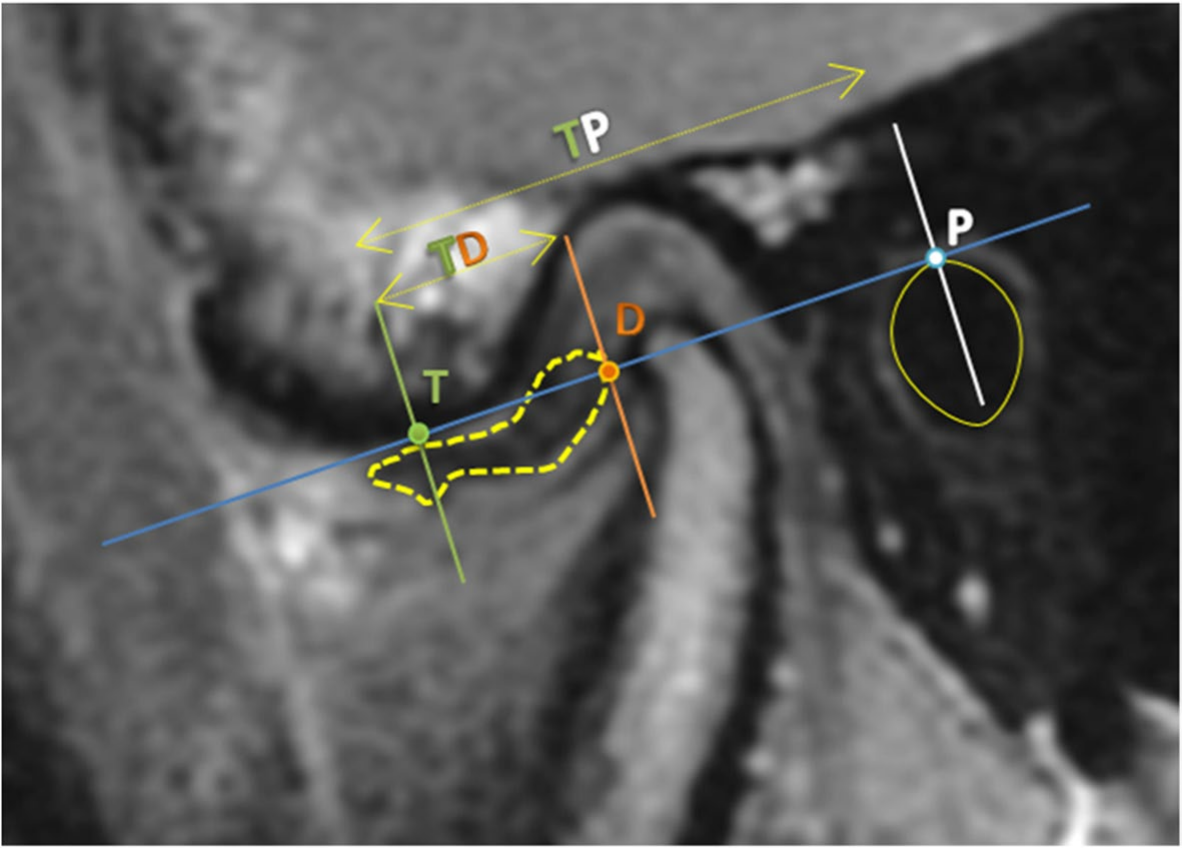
Application of Kurita et al. method on MRI for measuring the disc position
